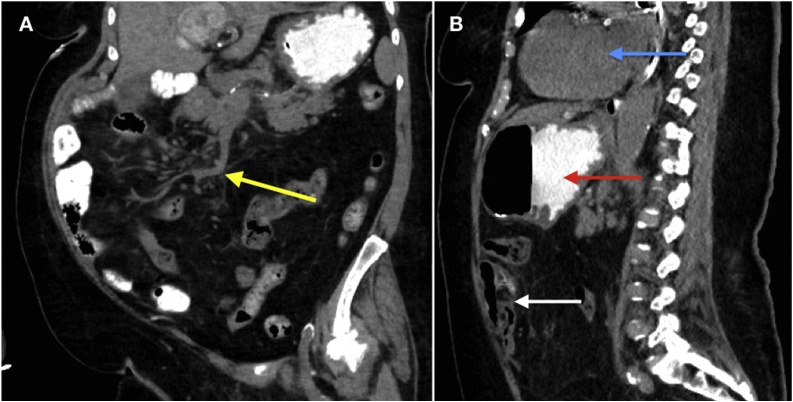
Coronal and sagittal images from non-contrast CT Abdomen and Pelvis obtained during postoperative period. A. (Coronal) Yellow arrow demonstrating root of mesentery that is no longer twisted. B. (Sagittal) New anterior and left hemi-abdomen position of ileocecal junction (white arrow). The stomach (red arrow) and heart (blue arrow) are seen supporting left-sided positioning. Appendix not visualized secondary to appendectomy.
